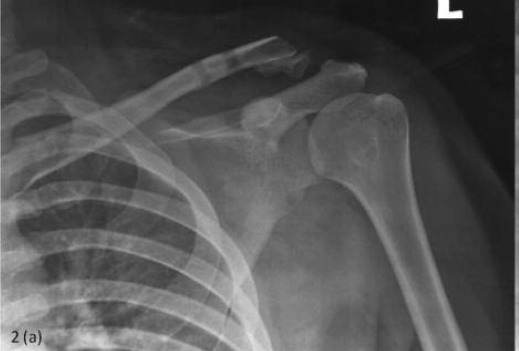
10 weeks post operative radiograph showing displaced
